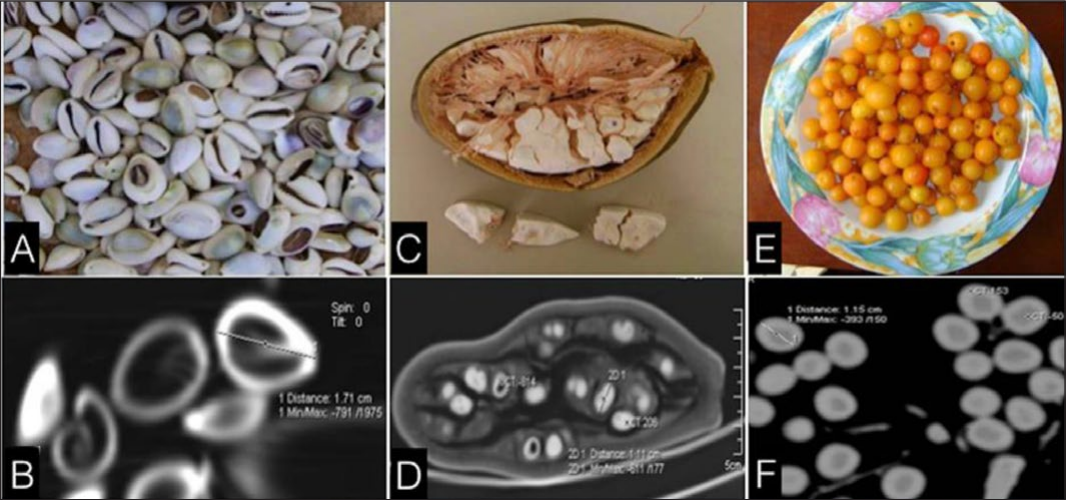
CT differential diagnoses of SS seeds. Photograph/CT of *Cowries*
